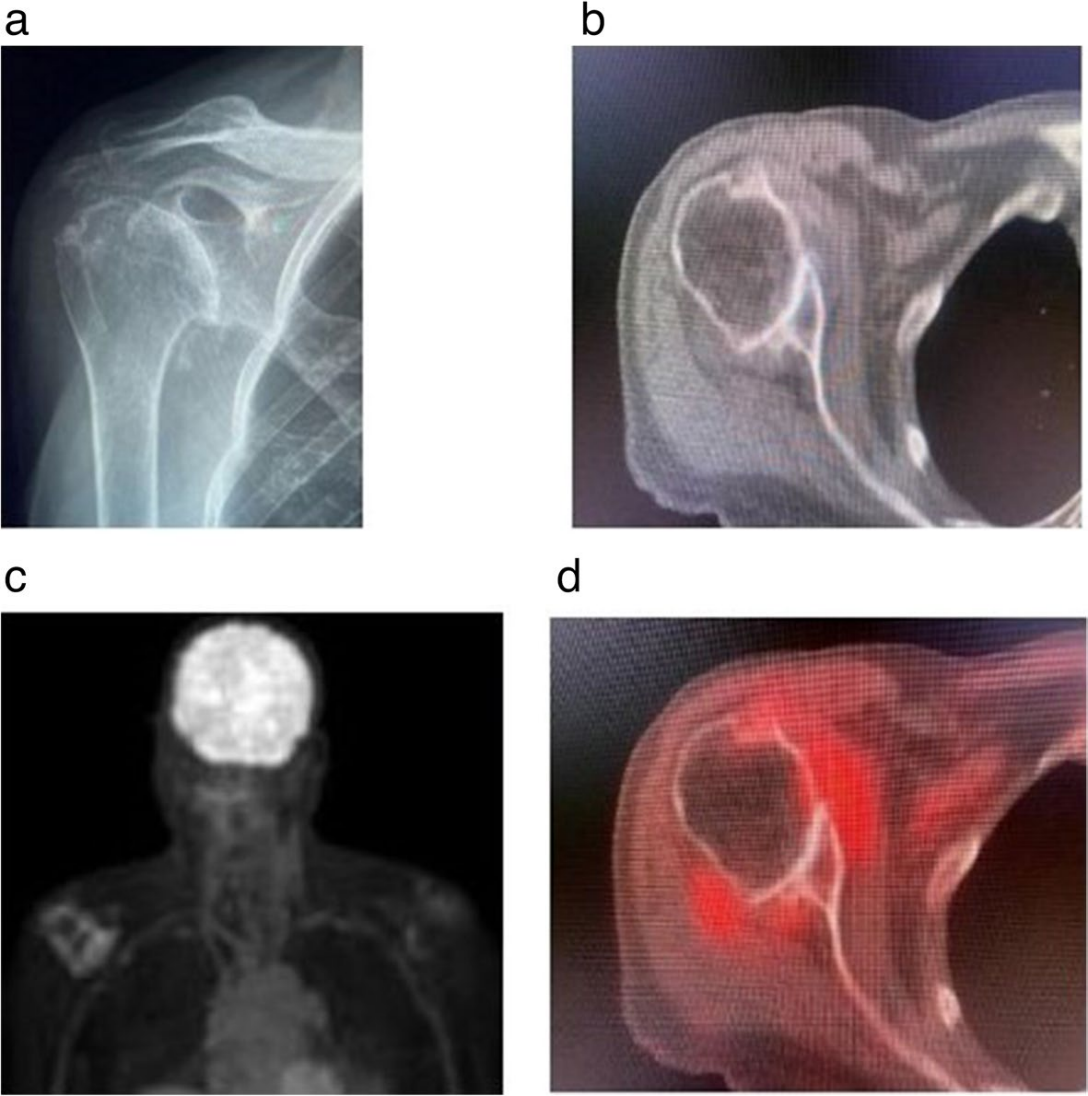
^18^F-FDG uptakes around right shoulder with osteoarthritis. a Frontal radiograph of the right shoulder shows glenohumeral joint OA with joint space narrowing and osteophyte formation. b Axial CT image at the level of the glenohumeral joints shows severe right glenohumeral joint space narrowing and osteophytosis, compatible with advanced OA. c MIP image from ^18^F-FDG PET-CT scan shows pathologic radiopharmaceutical accumulation around the right glenohumeral joint. d Fused axial and coronal PET-CT images show hypermetabolic activity along the periphery of the right glenohumeral joint in the expected location of the synovium and joint capsule, compatible with synovitis associated with OA
